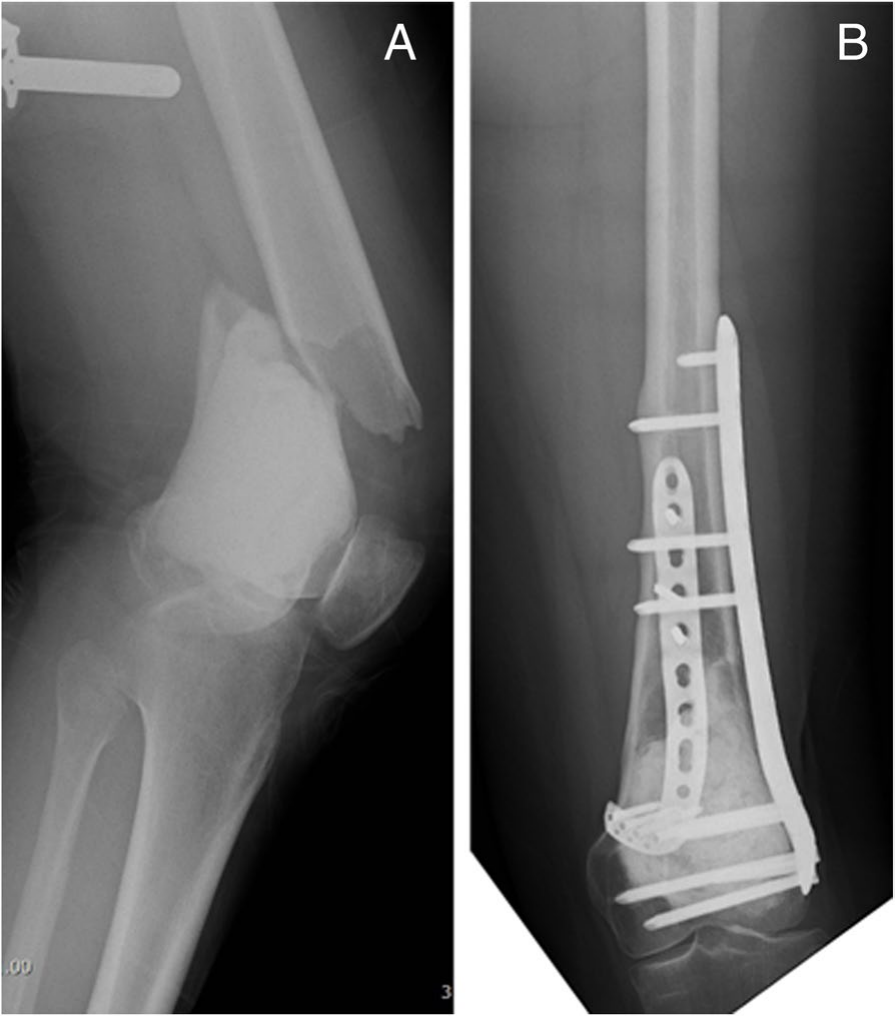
A, Radiography at the time of fracture (Case 10, Table 2). B, Radiography 3 years and 8 months after open reduction and internal fixation showed bone union
